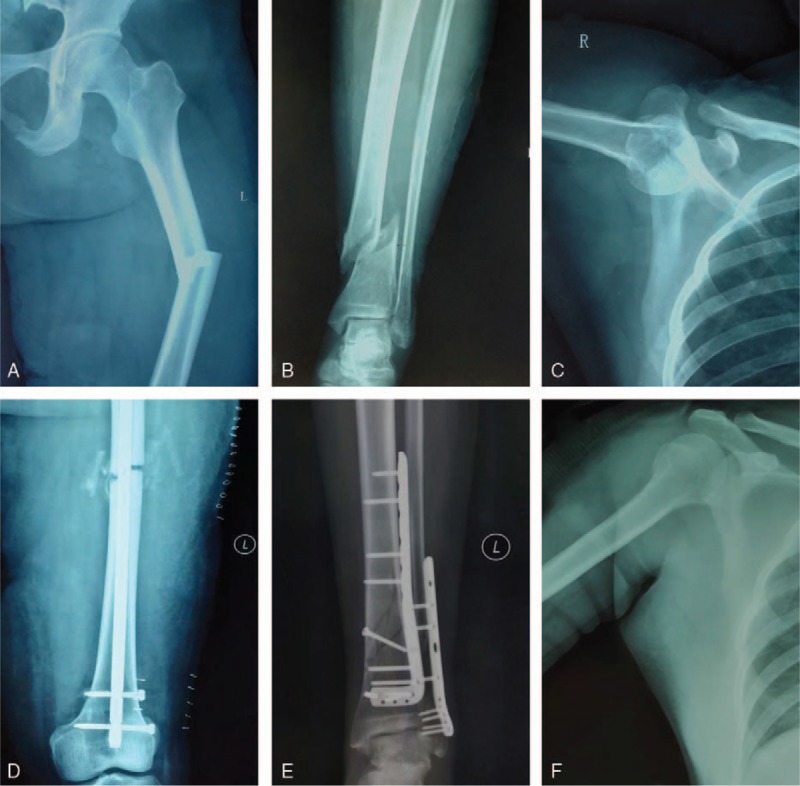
The preoperative and postoperative x-ray images of extremities and shoulder. (A) The femur was broken and two of the fractures formed an angle outwardly. (B) The tibia was fractured with the proximal fracture shifting to outside. The fibular fracture was not obvious. (C) This image shows anterior humeral head dislocation. (D) It showed the femoral shaft was fixed with intramedullary nail alone with bone grafting on broken ends and deformity corrected. (E) The tibia and fibula fractures were fixed with plates and screws, respectively. (F) The head of humerus returned to anatomical position after closed reduction.
